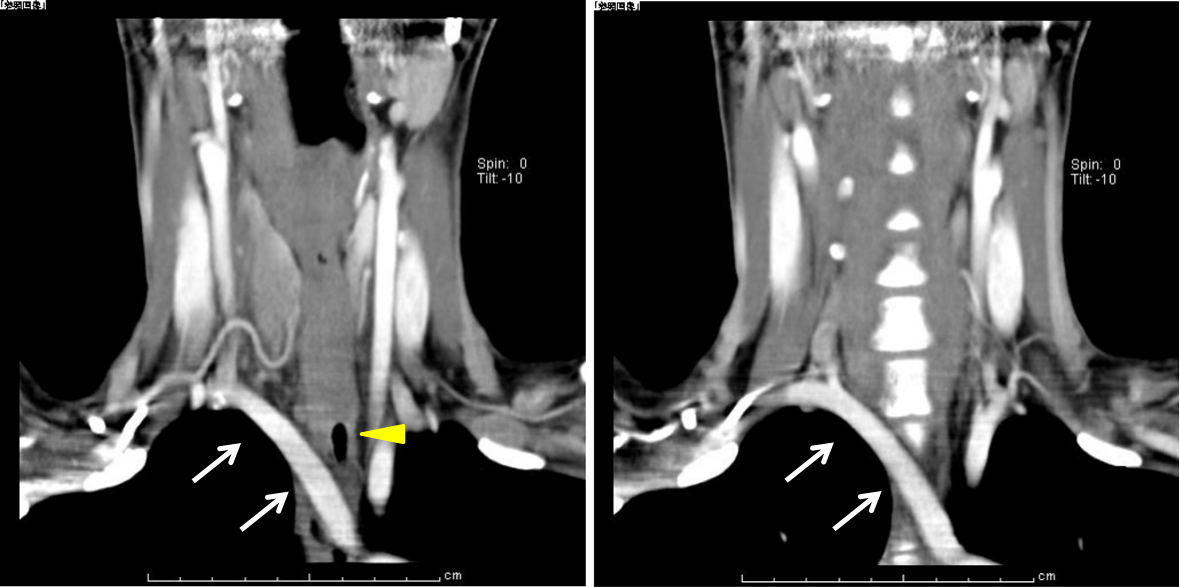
Preoperative enhanced CT scan of neck and chest of case 2. An aberrant right subclavian artery (*white arrow*) was found crossing behind the esophagus (*yellow arrowhead*)
